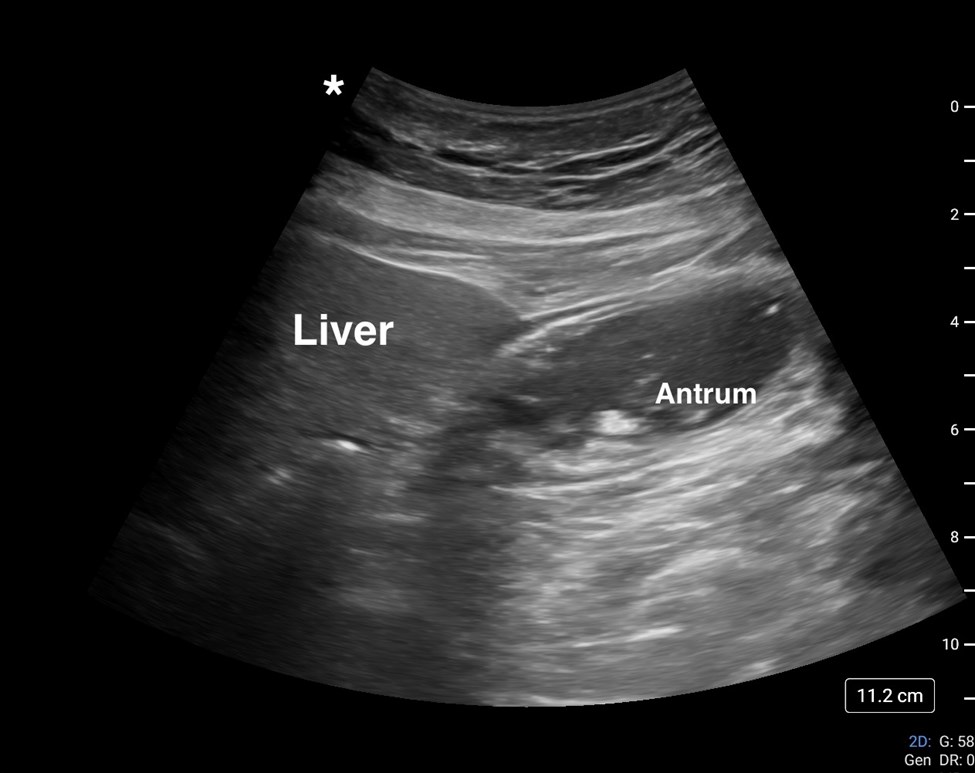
Gastric ultrasound image case #2
